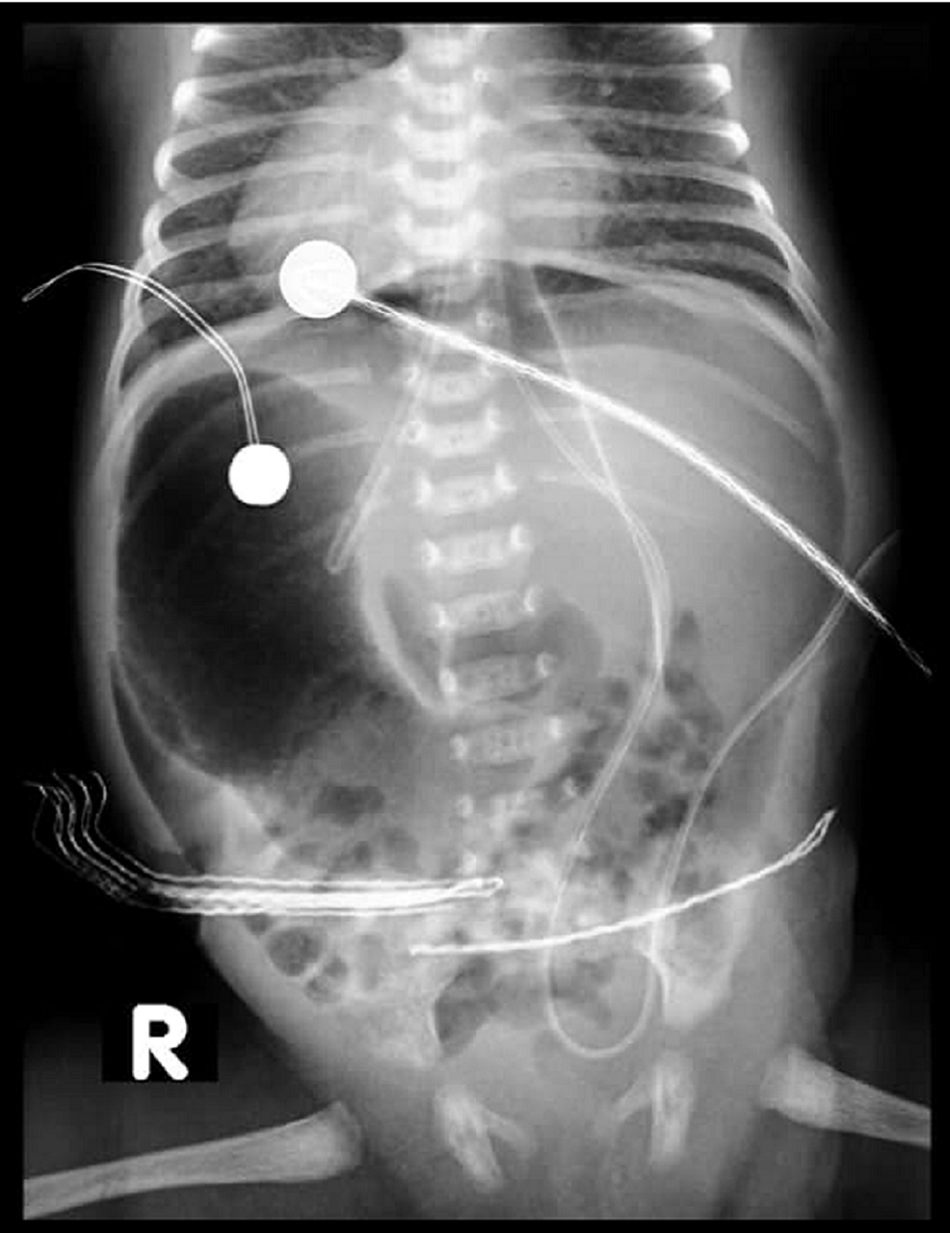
Abdomen postoperative X-ray showed megaduodenum and pneumoperitoneum.
